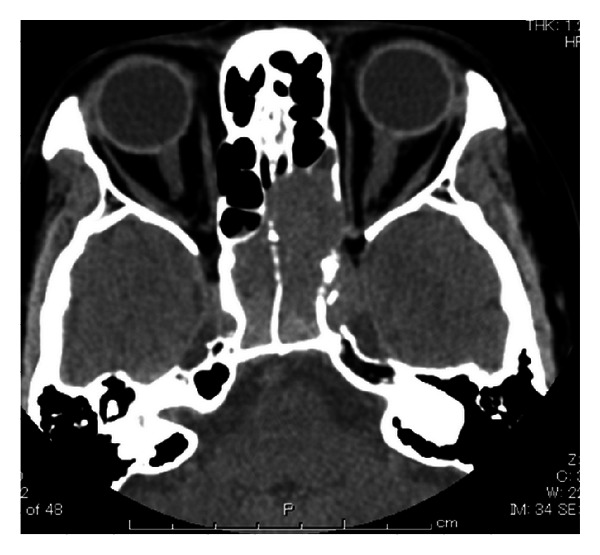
CT findings. In both the left and right sphenoid sinuses, soft tissue shadows accompanying skull base damage are apparent.
